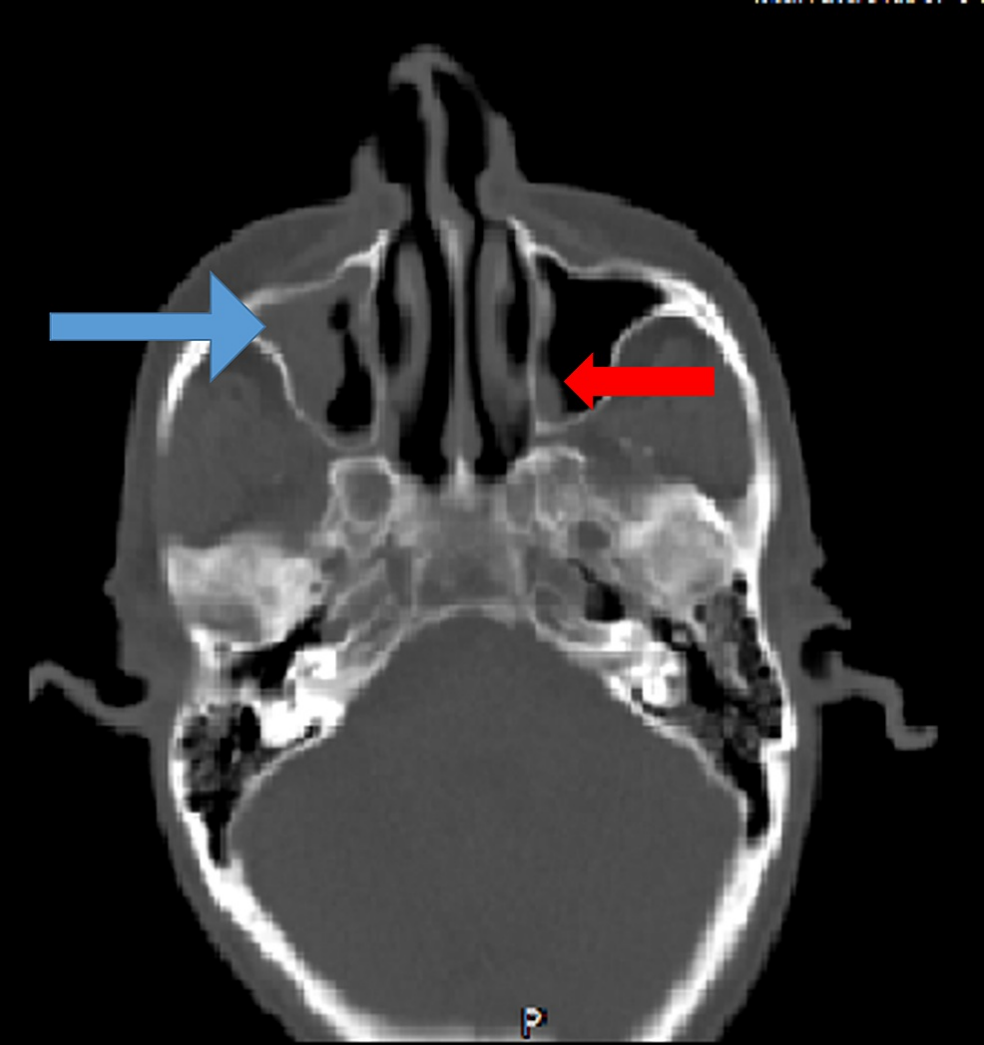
Axial CT head showing polypoidal mucosal thickening of right maxillary sinus (blue arrow) and focal thickening of left maxillary sinus (red arrow)
